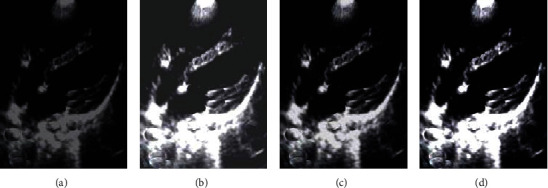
Demonstration of the noise reduction effect of three algorithms on echocardiography: (a) the original image; (b) the NLM algorithm; (c) the Bayes Shrink denoising algorithm; (d) the ONLM algorithm.
